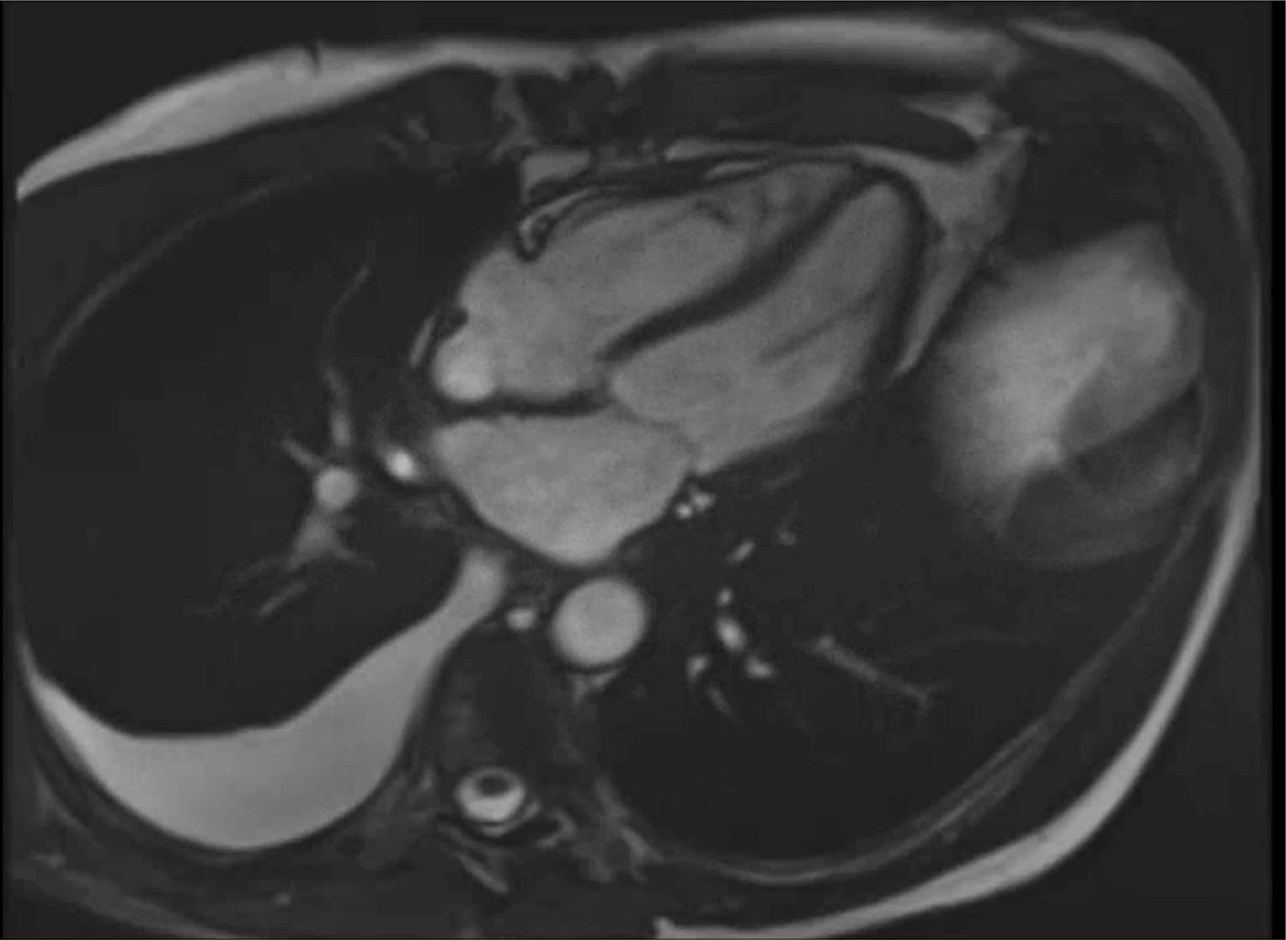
Pericardial constriction in RICD. Cardiac MRI demonstrating pericardial thickening and interventricular dependence in a patient with constrictive pericarditis
